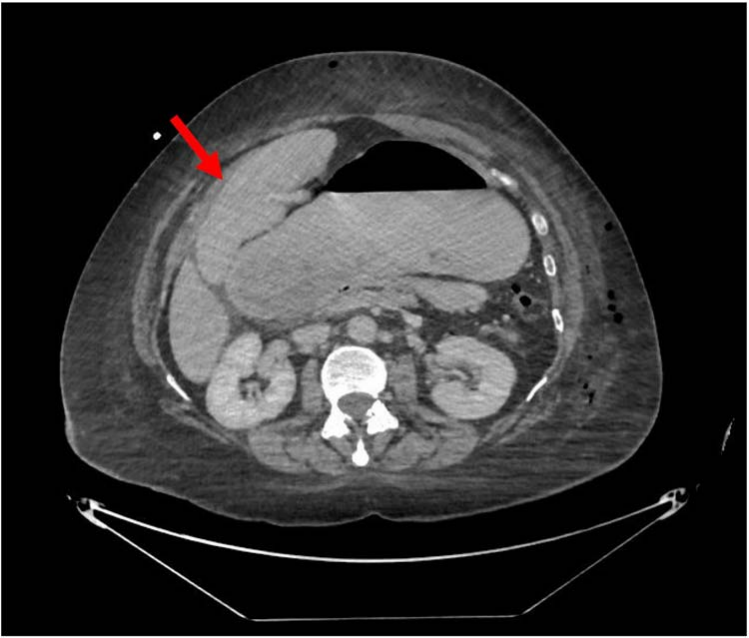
CT of the abdomen and pelvis (axial slice) showing gastric distention and the spleen positioned anteriorly in the right upper quadrant.
